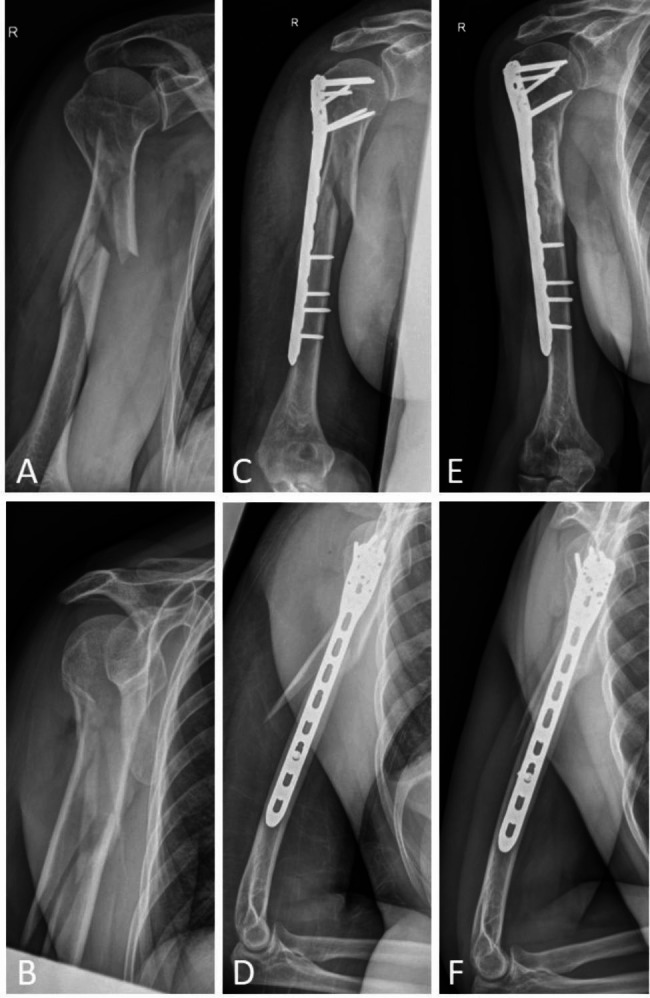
Case example of a 59-year old woman after a stair fall. A/B. The multifragmentary diaphyseal fracture with extension into the proximal metaphysis (AO 12C2(i)) was bridged with a minimally invasive lateral plate osteosynthesis the same day. C/D. Postoperative radiographs show good fracture alignment and plate position. E/F. At 12-month follow-up radiographs demonstrate bone healing
